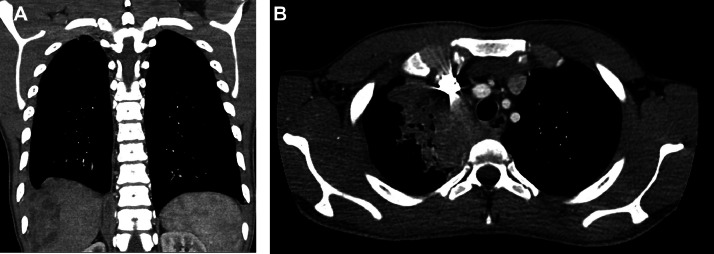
Chest CT scan, in coronal (A) and sagittal (B) sections. Imaging revealing large right upper lung cavitary mass with bilateral pulmonary nodules and masses. Nodularity of the right pleura is also seen, representing possible metastatic disease or complex pleural collection in the setting of TB. Adjacent subcapsular lobulated lesions were noted in the right hepatic lobe.
